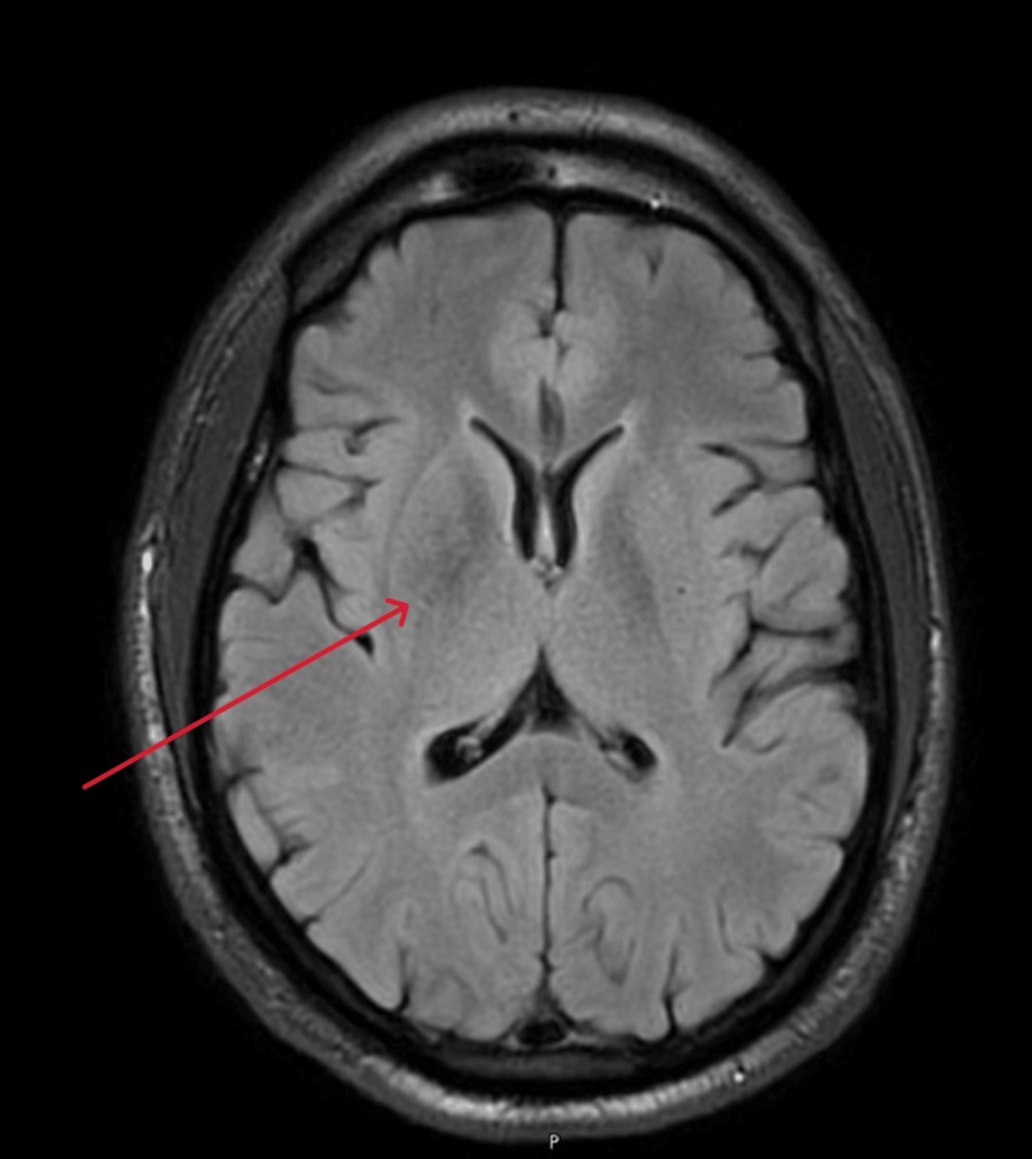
Magnetic resonance imaging/FLAIR revealing subtle hypointensity noted in the right basal ganglia (red arrow) FLAIR: fluid-attenuated inversion recovery
